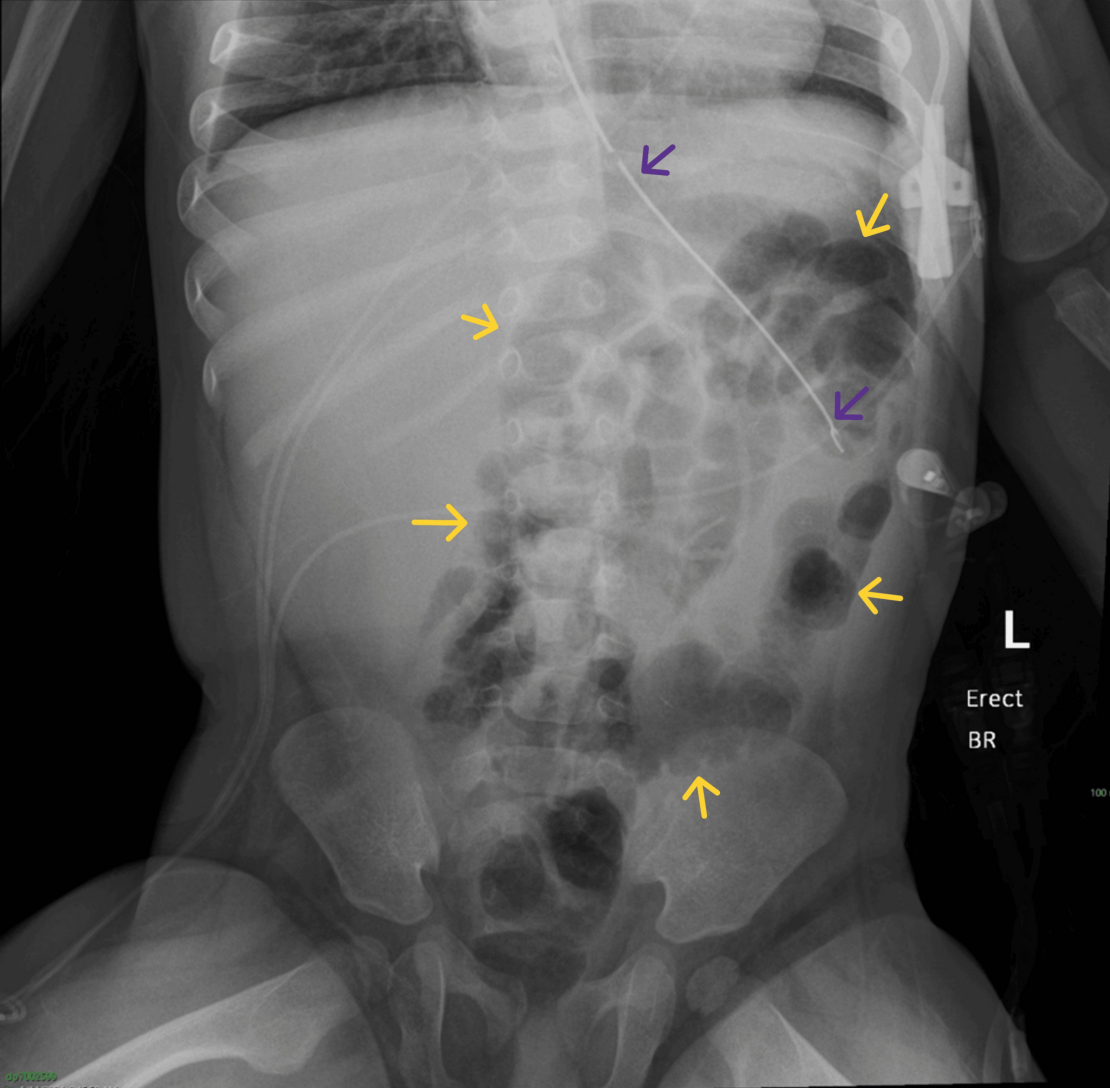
One-week postintervention abdominal radiograph, AP view: complete resolution of previously seen abnormal large bowel gaseous distension. There is a normal, non-obstructive large bowel gas pattern (yellow arrows). Note the persistent presence of the nasogastric tube (purple arrows) within the stomach for continued decompression. AP = anteroposterior
